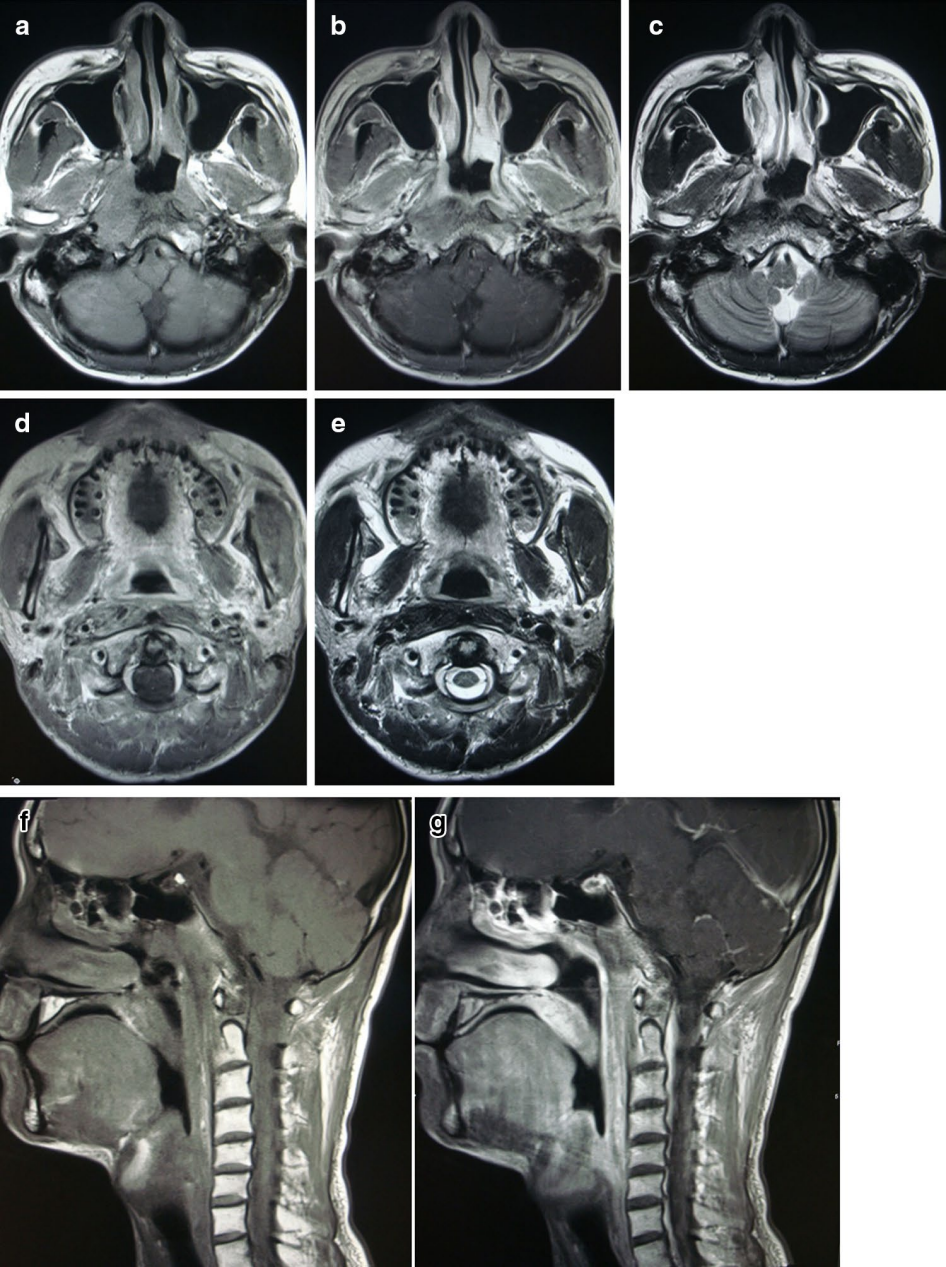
MR imaging of the NPC patient at 3 months after treatment. T1-weighted axial MR image of the nasopharynx a without contrast, b with contrast, and c T2-weighted axial MR image of the nasopharynx show edema signal of the nasopharyngeal mucosa but no mass. T1-weighted axial MR image d with contrast of previous occipital lymph node level and e T2-weighted axial MR image of previous occipital lymph node level show normal structure now. T1-weighted sagittal MR images f without contrast and g with contrast reveal no mass as well
